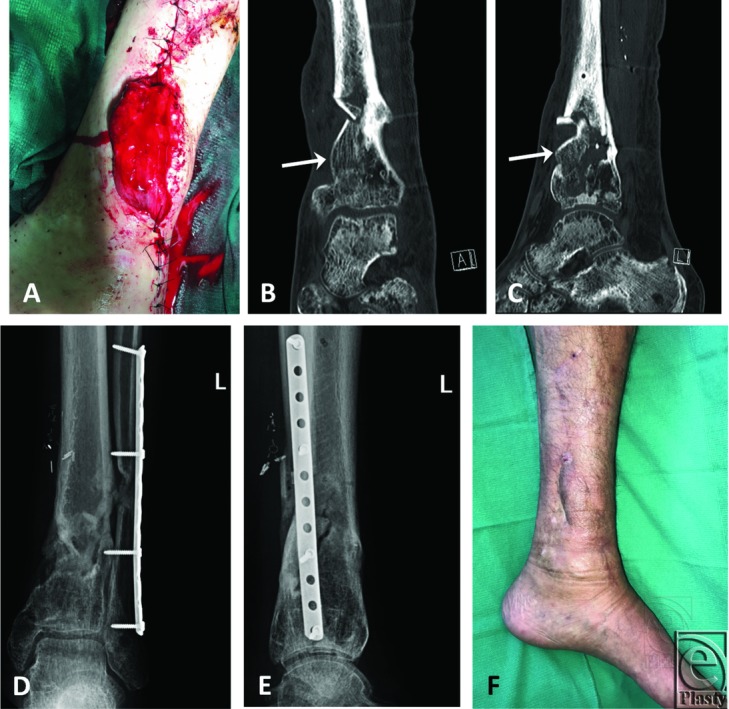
Immediate postoperative view of the chimeric myo-osseous MFC flap (a), AP (b), and lateral (c) computed tomographic scans of the MFC flap (arrow) at 3 months postoperatively, AP (d) and lateral (e) plain radiographs of the flap at 9 months postoperatively showing good bone healing, and a final photograph of the healed flap at 9 months postoperatively (f). MFC indicates medial femoral condyle; AP, anteroposterior.
